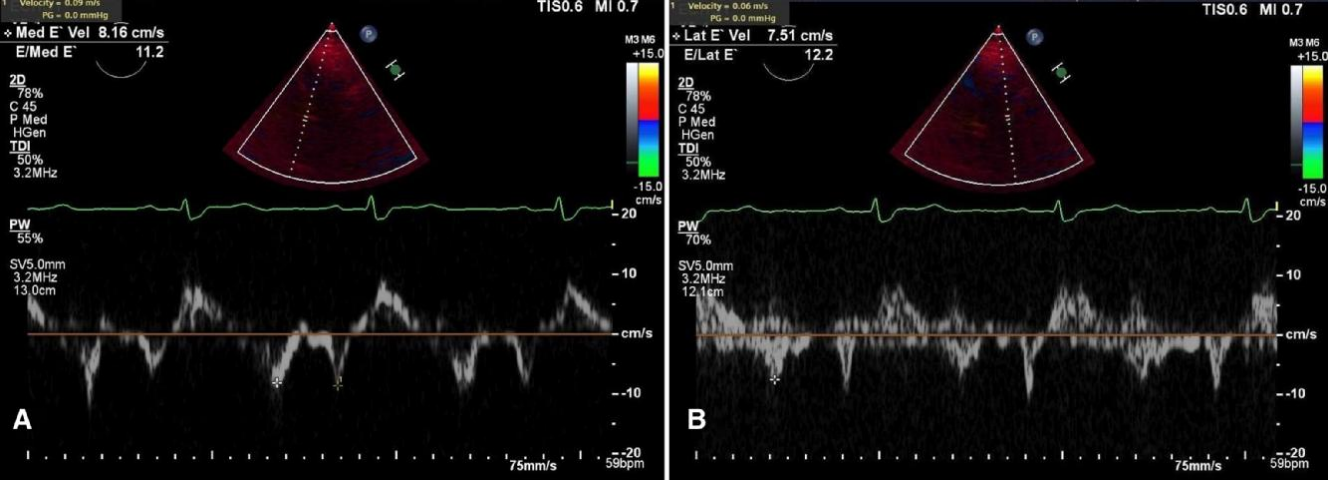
Transthoracic echocardiogram. (*A*) Medial mitral annular velocity and (*B*) lateral mitral annular velocity demonstrating *annulus reversus*.
